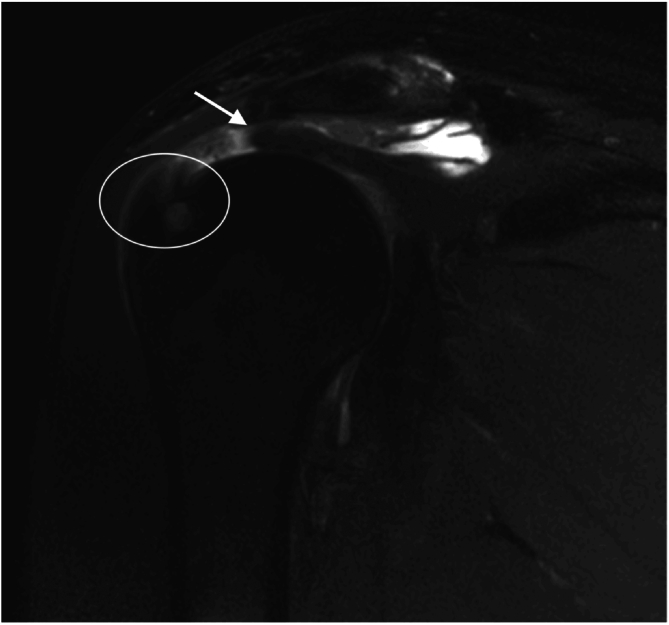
Right shoulder MRI, T2-weighted fat-saturated coronal view of a traumatic tendon shortening 2 months after a fall while hiking. Observe the residual stump at the greater tuberosity^45^ (B2^31^ (midsubstance) lesions) (circle). The supraspinatus tendon has shortened (arrow). (MRI, magnetic resonance imaging.)
